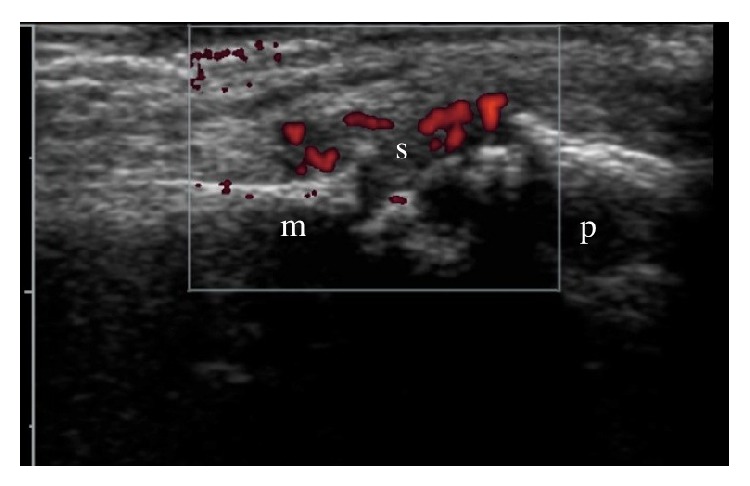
Longitudinal sonographic view of the right second metacarpophalangeal joint (m: metacarpal, p: proximal phalanx) in a patient with rheumatoid arthritis showing synovitis/effusion (s) with power Doppler flow (red).
